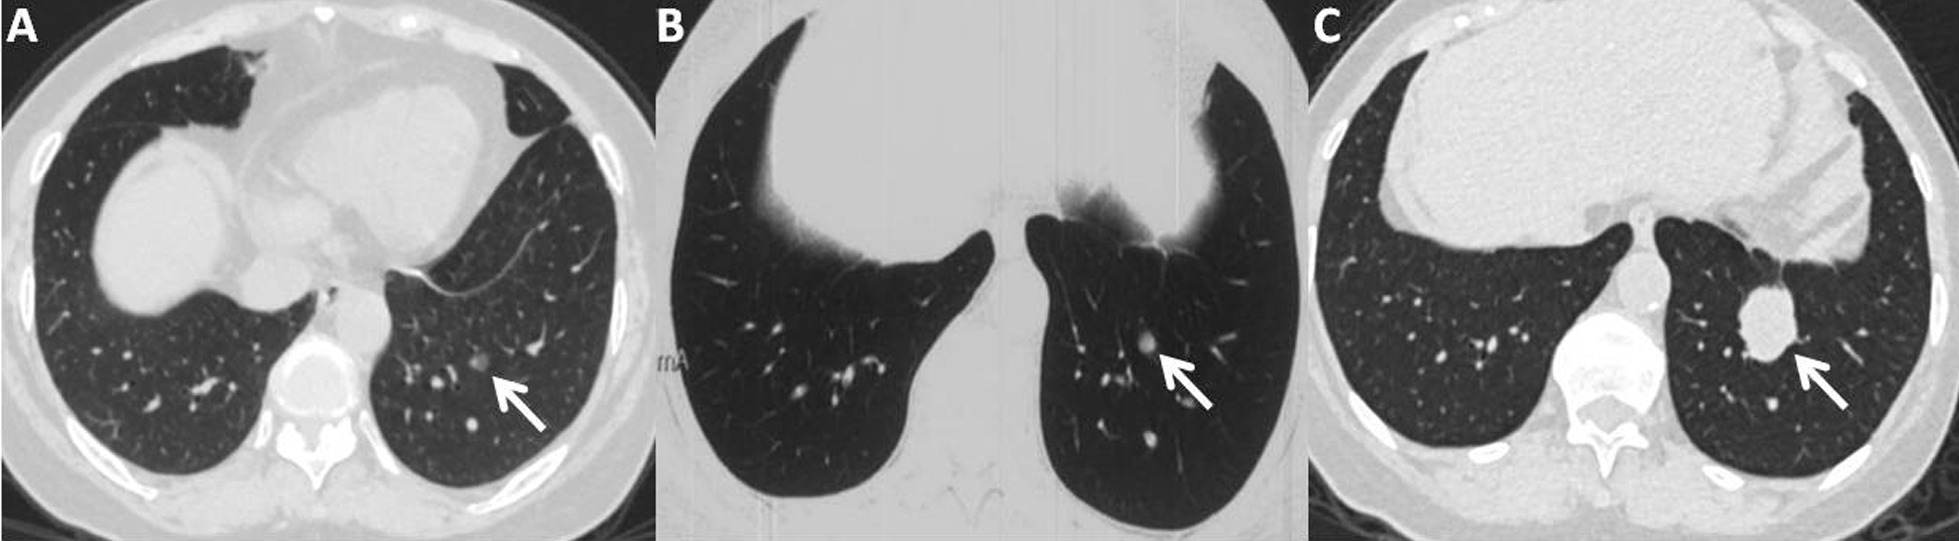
A The chest CT on 29th December, 2019 revealed a 0.8 cm × 0.6 cm mixed GGO lesion with a distinct boundary on her left lower lobe, which comprised 3 mm subsolid components; B The second chest CT on 15th September, 2020 showed that there was no significant change in the size of this nodule, but revealed a larger solid component within the mixed GGO lesion; C The third chest CT on 19th April, 2021 showed a 3.1 cm × 2.9 cm completely solid nodule with discriminated boundary and sign of lobulation
